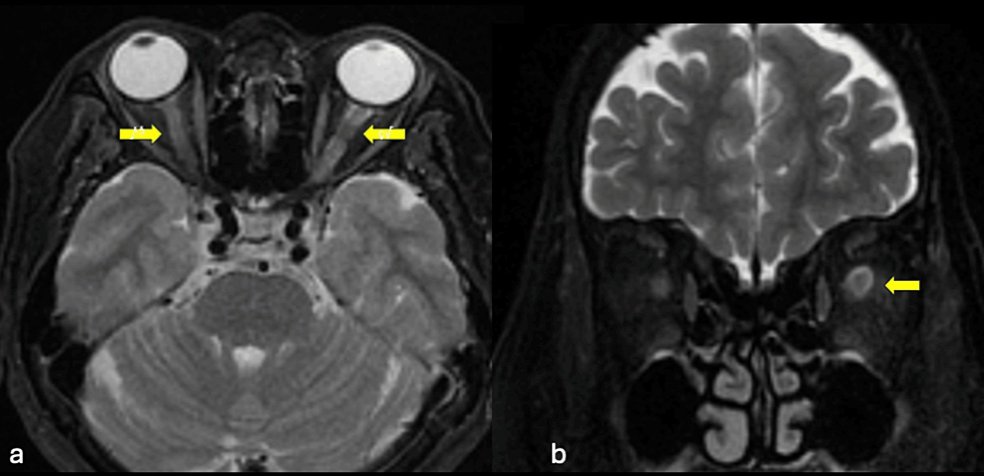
T2-weighted image axial view showed presence of bilateral tram-track sign (yellow arrow in a), and doughnut sign in the coronal view (yellow arrow in b).
